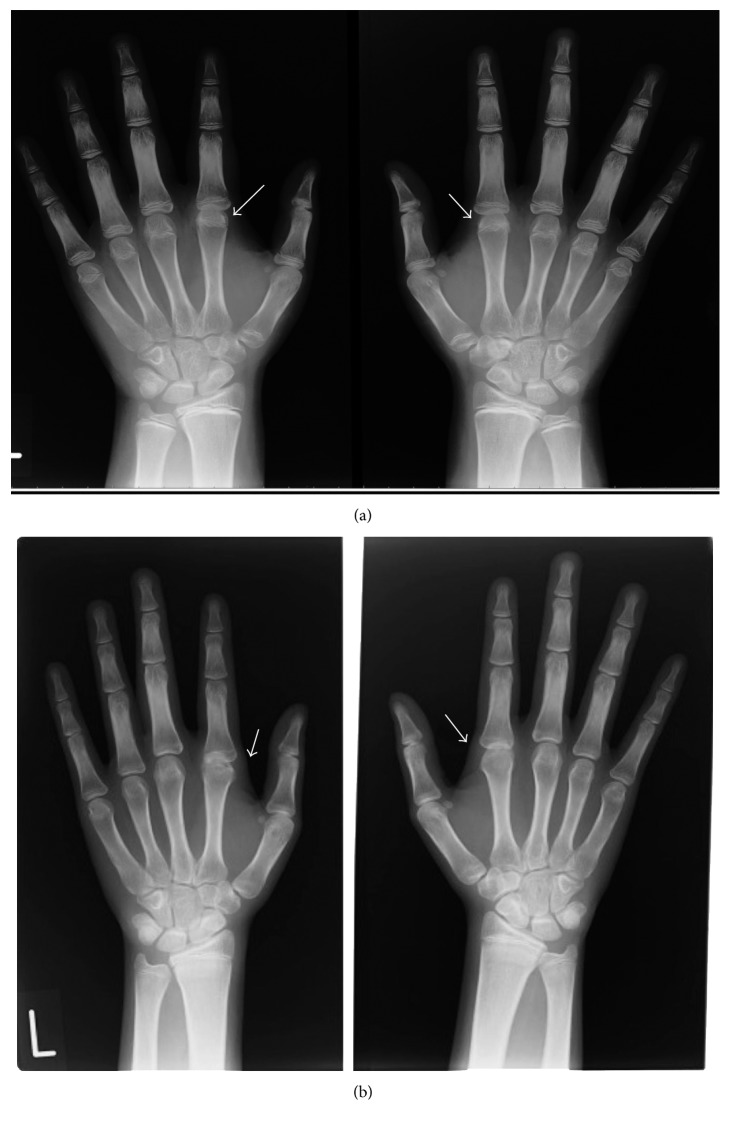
Radiography of both hands. (a) In September 2014, at the initiation of adalimumab when the patient was 12 years and 1 month of age. (b) In September 2016, after 2 years of adalimumab treatment when the patient was 14 years and 1 month of age. Narrowing of the metacarpophalangeal joints of both index fingers was observed when adalimumab was started (a), and more progressive joint space narrowing existed under treatment with adalimumab, whereas insignificant bone erosion was observed (b).
